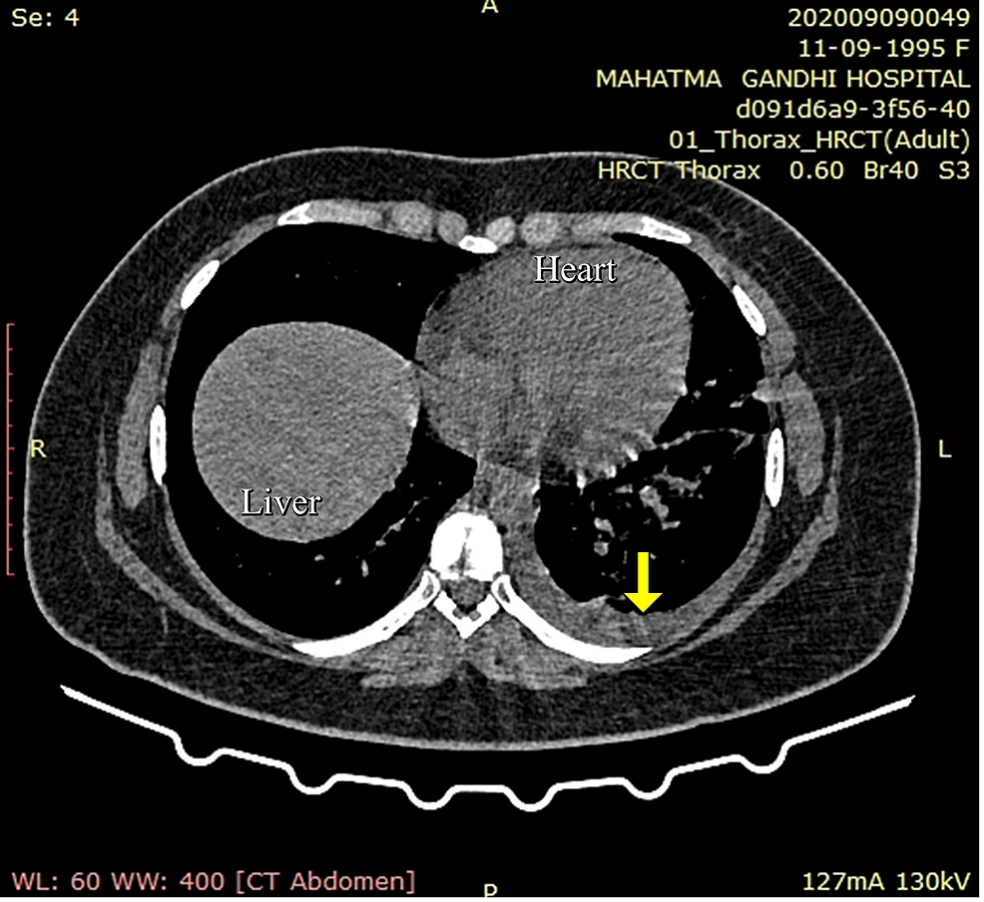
HRCT of the chest mediastinal window (axial section) showing left pleural effusion (solid yellow arrow). HRCT, high-resolution CT
